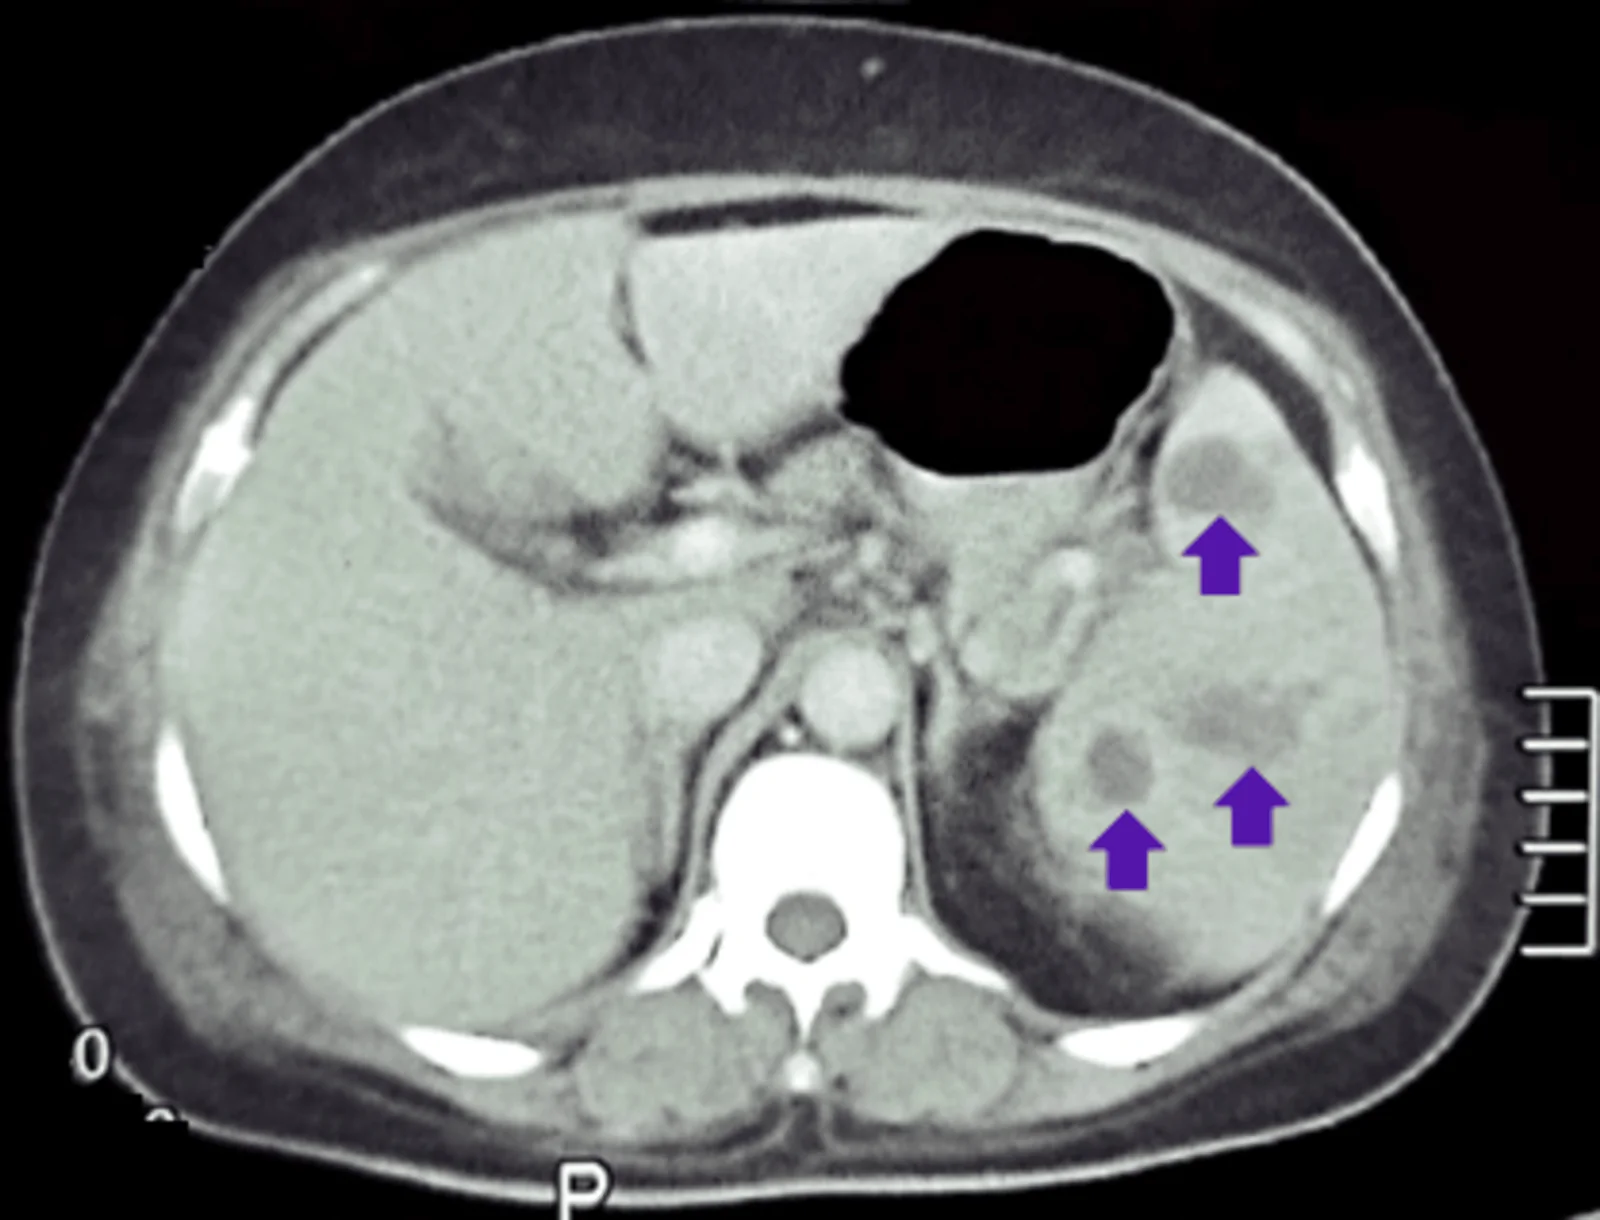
Abdominal CT scan showing multiple splenic abscesses
